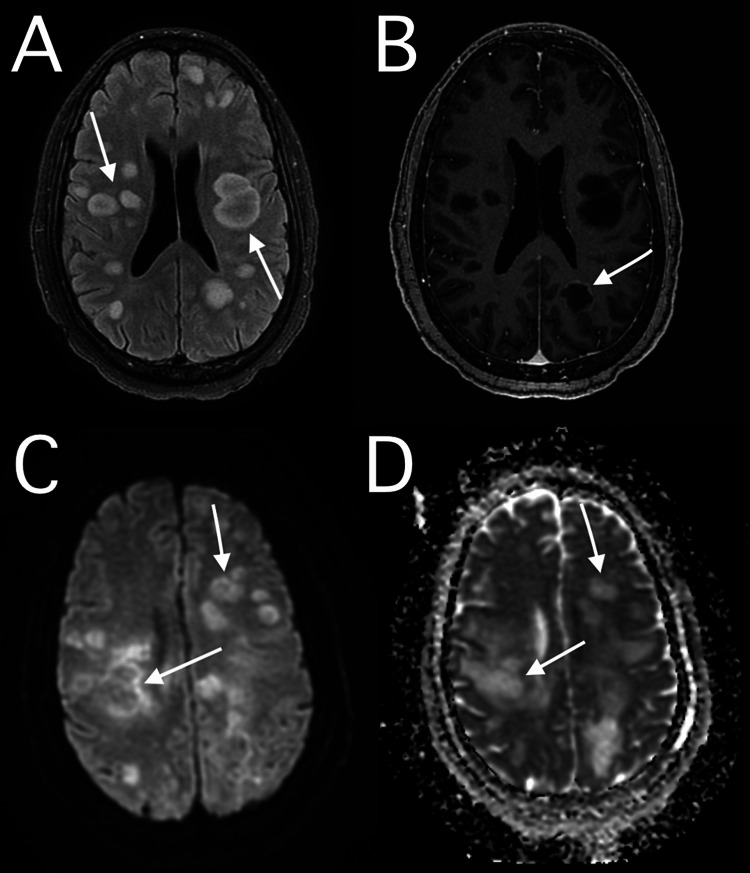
Magnetic Resonance Imaging (MRI) of the Brain MRI of the brain was performed with and without contrast which showed multifocal and confluent hyperintense lesions (arrows) in the bilateral subcortical and deep white matter on T2-weighted fluid-attenuated inversion recovery phase (A). On the T1-weighted post-contrast sequence (B), these lesions showed interrupted peripheral enhancement (arrow). Diffusion-weighted images (C) with corresponding apparent diffusion coefficient map (D) showed central elevation of diffusion coefficient with a peripheral ring of reduced diffusion coefficient (arrows).
